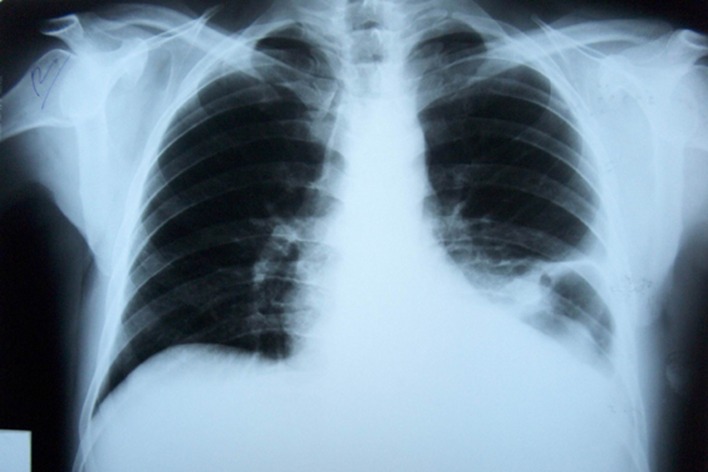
Pre-treatment PA chest radiograph of the case 2: a large pleural effusion evident in the left hemithorax was seen.
